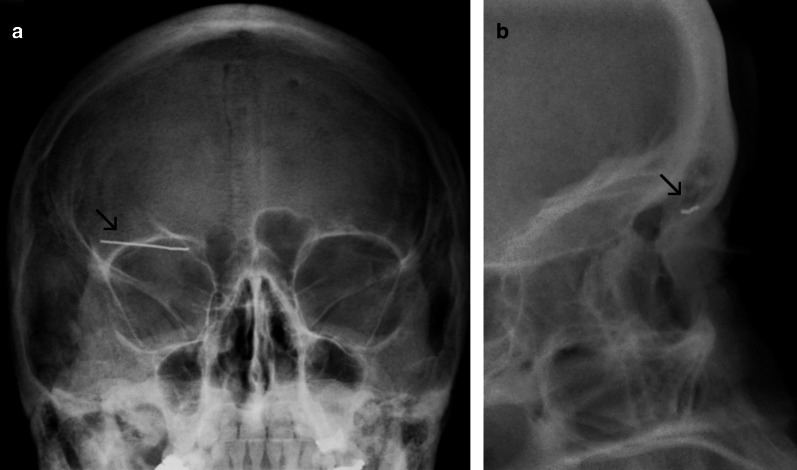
Paper clip. Anteroposterior (a) and lateral (b) radiographs showing a linear radiopaque structure in projection of the upper eyelid (arrow)
